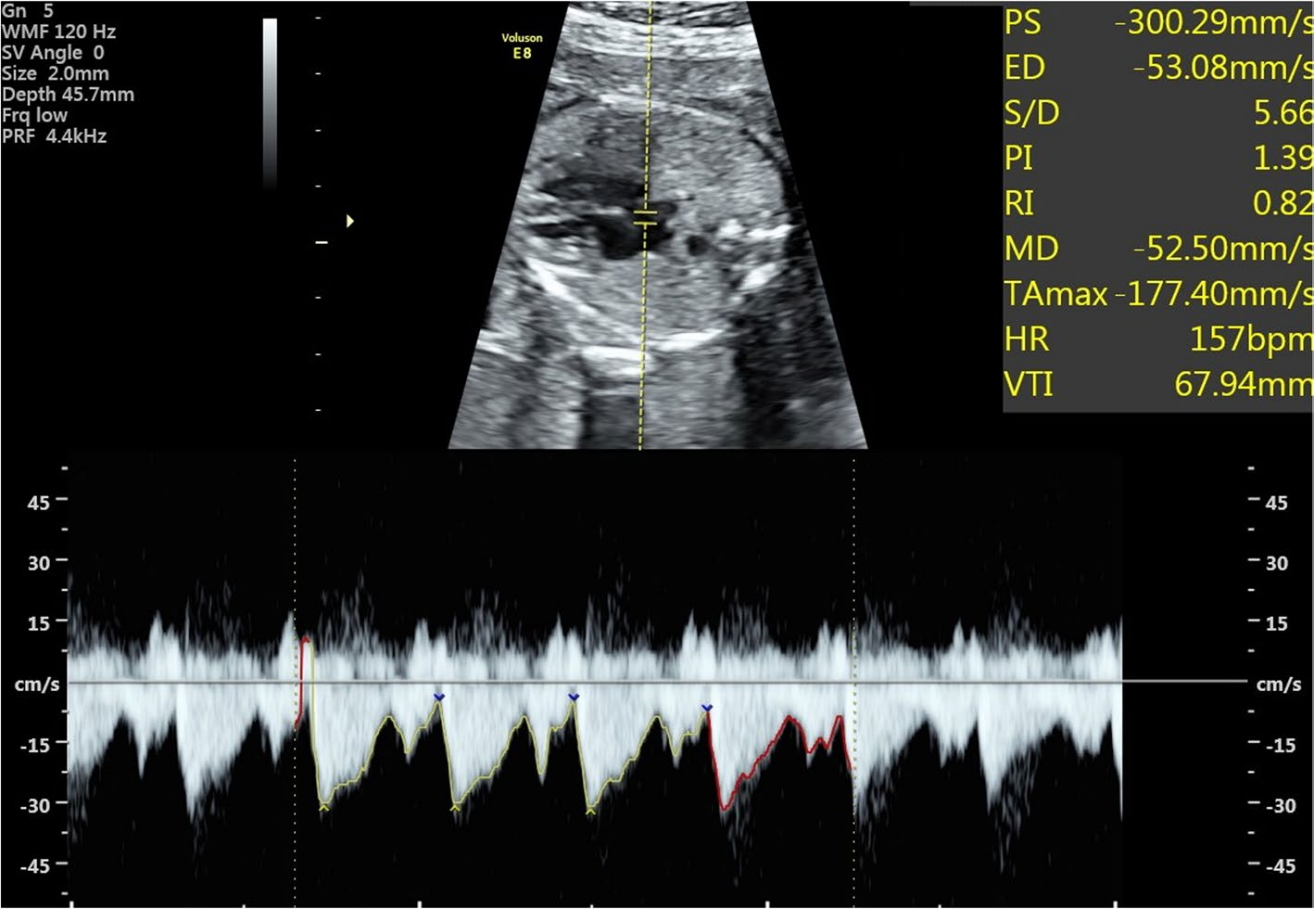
Measurement of the foramen ovale blood velocity waveforms. The Doppler sample volume is located within the left atrium and is parallel to the transarterial flow. The transforaminal flow is bidirectional, with right-to-left flow predominating
